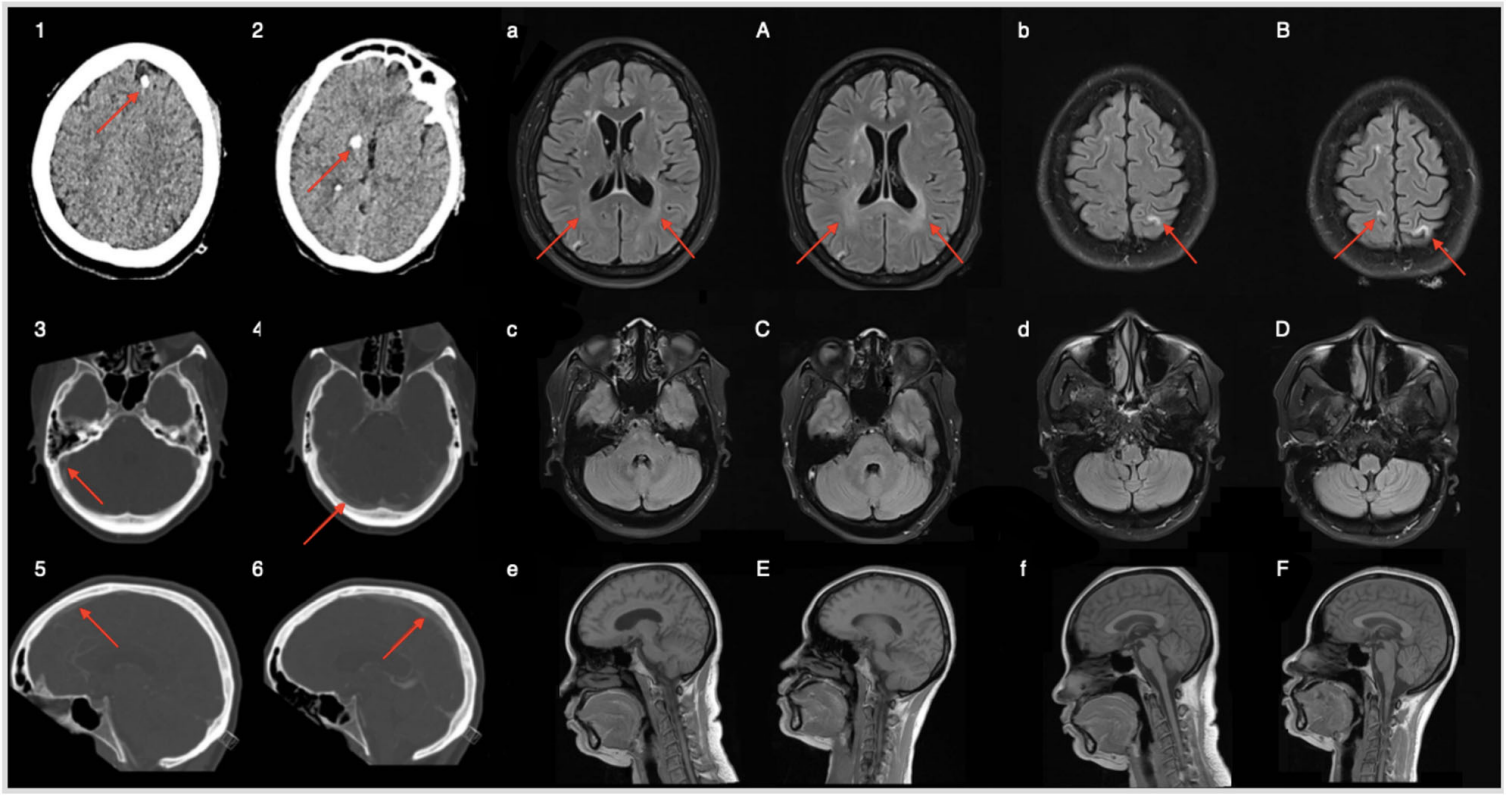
Brain CT on admission (panels 1–2) shows sequellar macro calcification of former neurotoxoplasmosis episodes (red arrows). Panels (3–6) refer to brain CT with angiography (venous phase); thrombosis of superior sagittal and right transverse sinus. Brain MRI realized 6 months before admission (a–f) and on admission (A–F). White matter hyperintensities in T2 FLAIR account for CSF HIV escape related progressive neuroinflammation. Absence of lesions in the posterior fossa.
